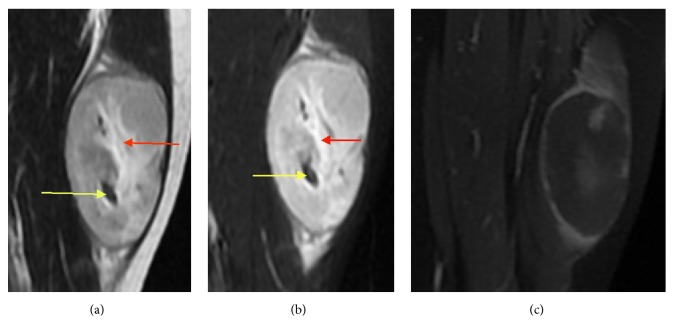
A 16-year-old female with IMT of the left thigh. (a) An oval-shaped soft tissue mass with short T1 and (b) long T2 signal was observed, with associated hemorrhage (red arrow) and vascular lesions (yellow arrow). (c) An enhanced MRI scan revealed rim enhancement.
